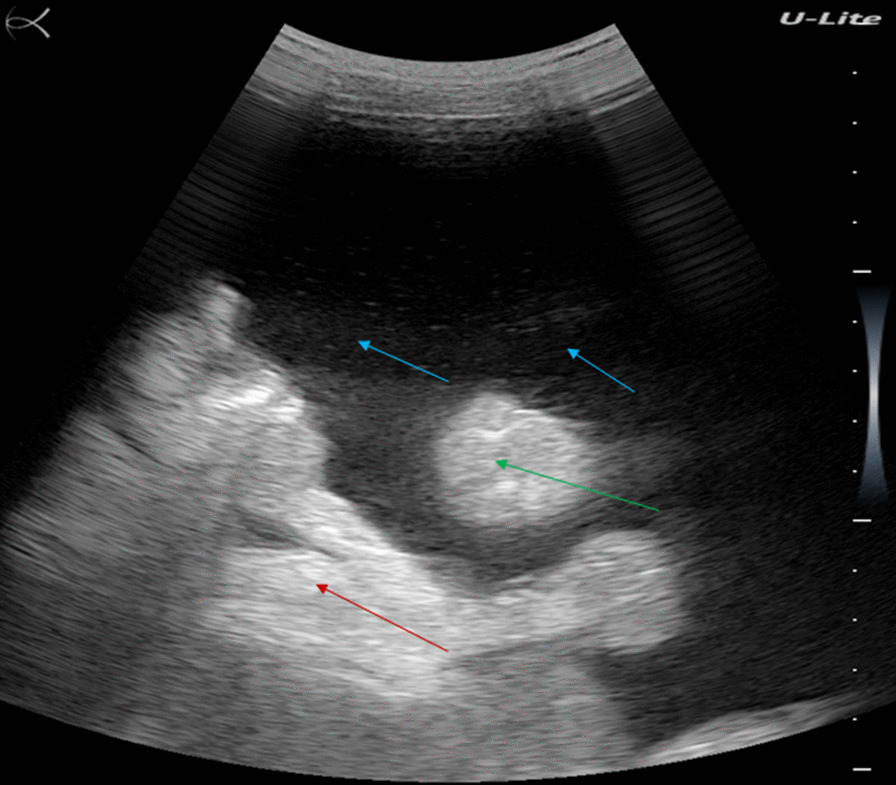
Ultrasound examination of patient #2, showing shrunken cirrhotic liver with distorted architecture, nodular surface, and heterogeneous echotexture of the parenchyma (red arrow), regenerating nodules (green arrow), and ascites (blue arrow)
